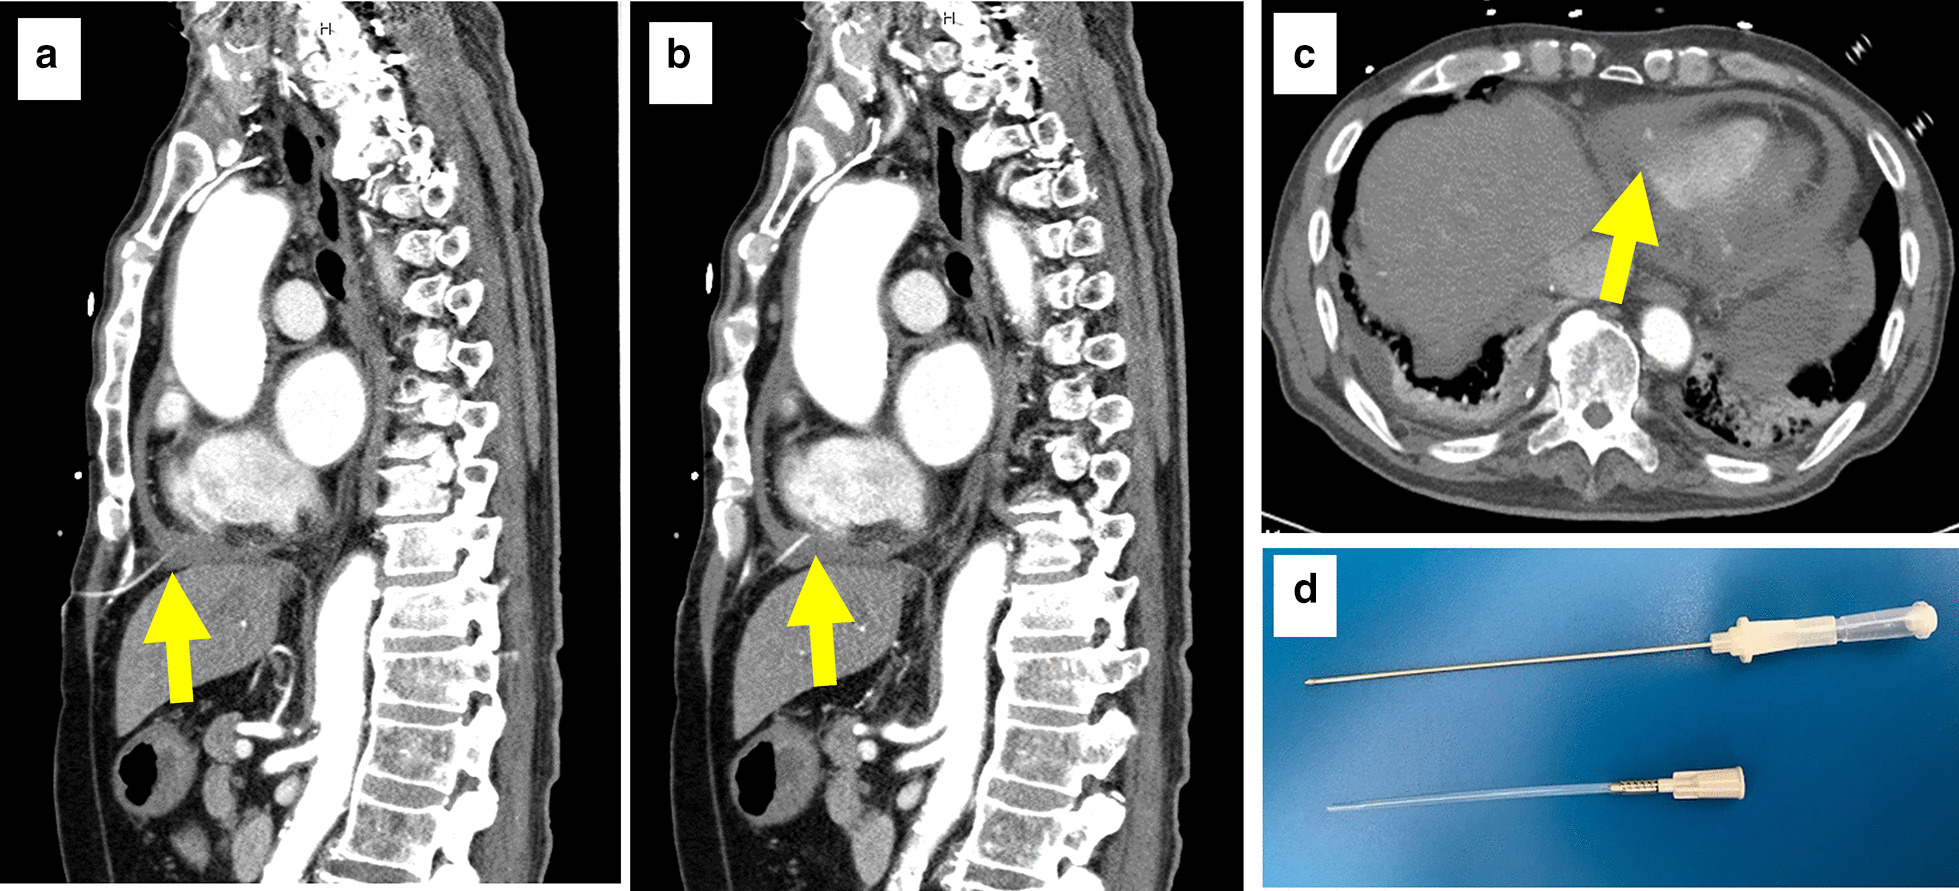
Pericardiocentesis and puncture needle. Computed tomography shows the tip of the outer cannula of the puncture needle in the pericardial cavity (a–c). Needle used for pericardiocentesis (d)
